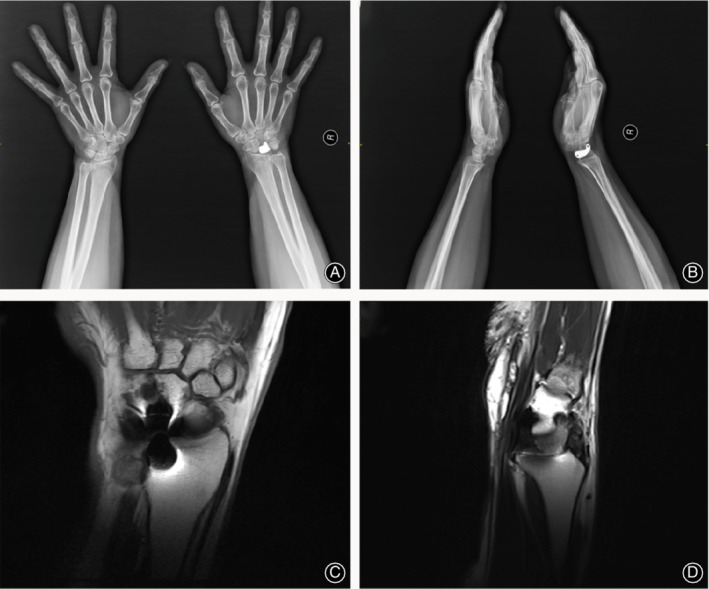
Views of the position of the 3D printing lunate and articular surface of radium from Fig. 1 at 30‐month follow‐up. (A, B) (X‐ray) show no dislocation nor subluxation of the prosthesis; (C, D) (MRI) show no degenerative change observed on the articular surface of the radium.
